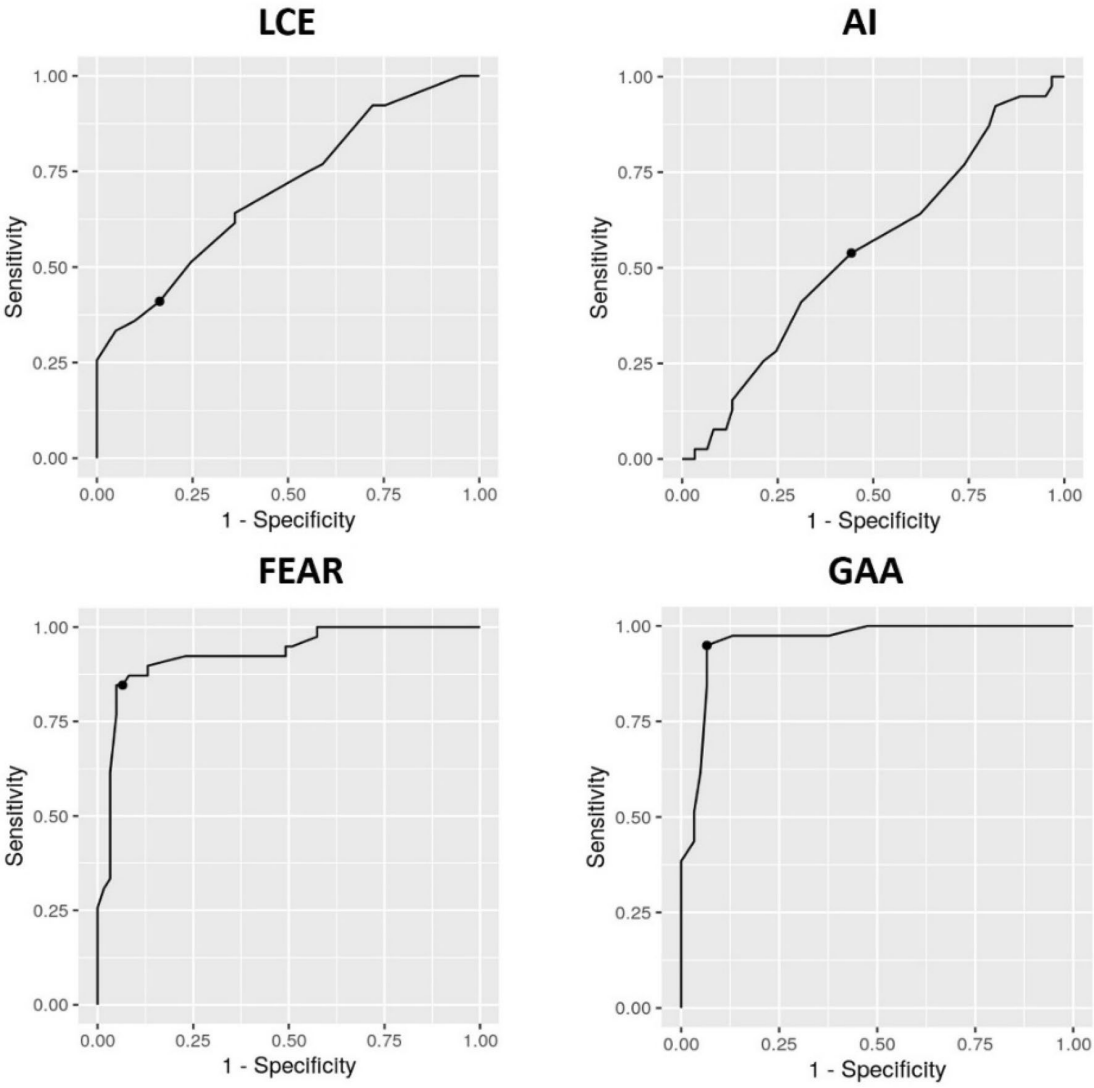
Simultaneous receiver operating characteristic (ROC) curves for radiographic parameters. *AI* acetabular index, *FEAR* Femoro-epiphyseal Acetabular Roof, *GAA* Gothic Arch Angle, *LCEA* Lateral Center Edge Angle.
